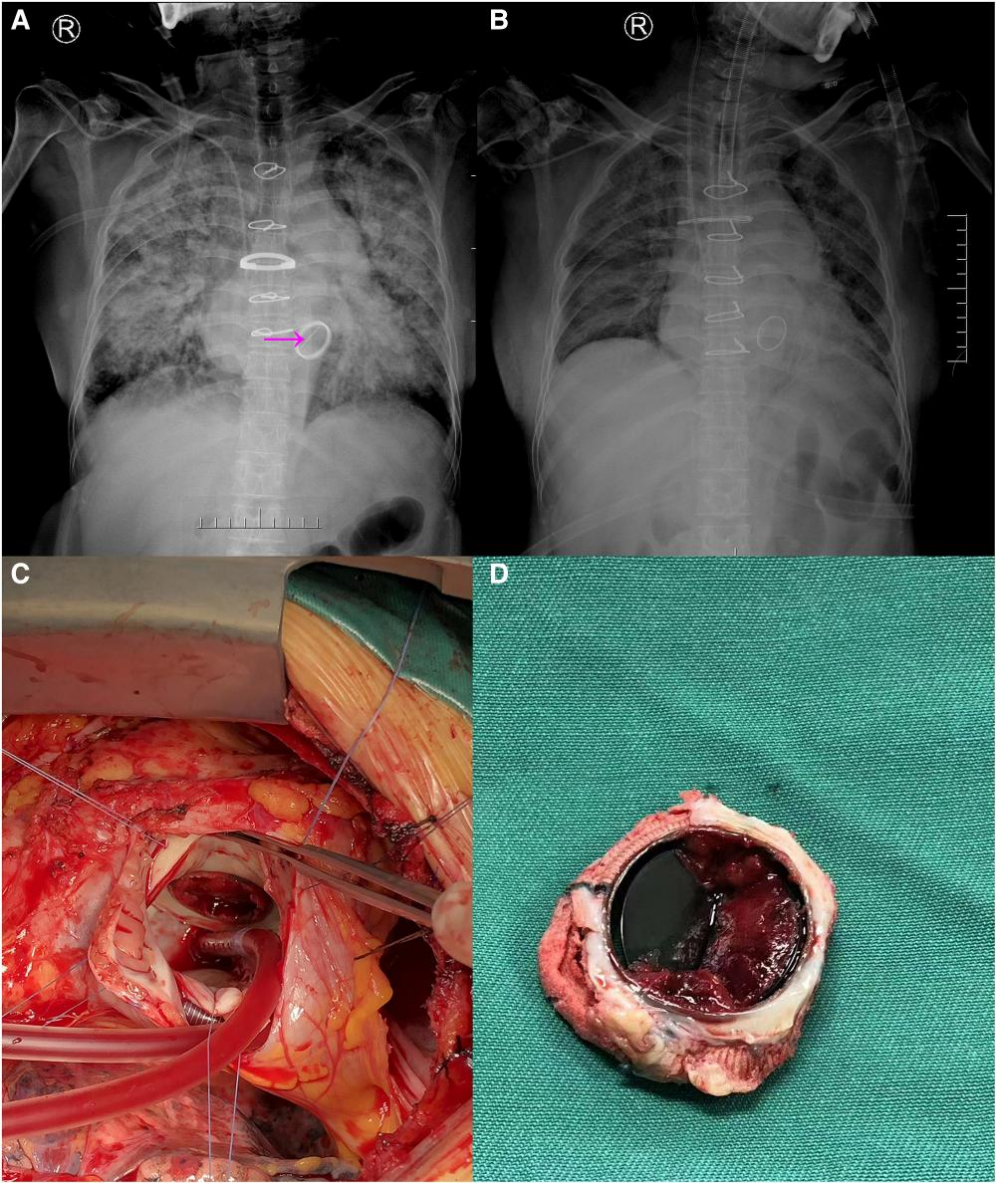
Imaging and surgical findings of acute prosthetic mitral valve obstruction. (*A*) Admission chest X-ray reveals the ‘frozen leaflet sign’ (arrow) and pulmonary venous congestion. (*B*) Postoperative chest X-ray shows resolution of the radiopaque shadow. (*C* and *D*) Intraoperative photographs confirming thrombus causing the valve leaflet obstruction.
